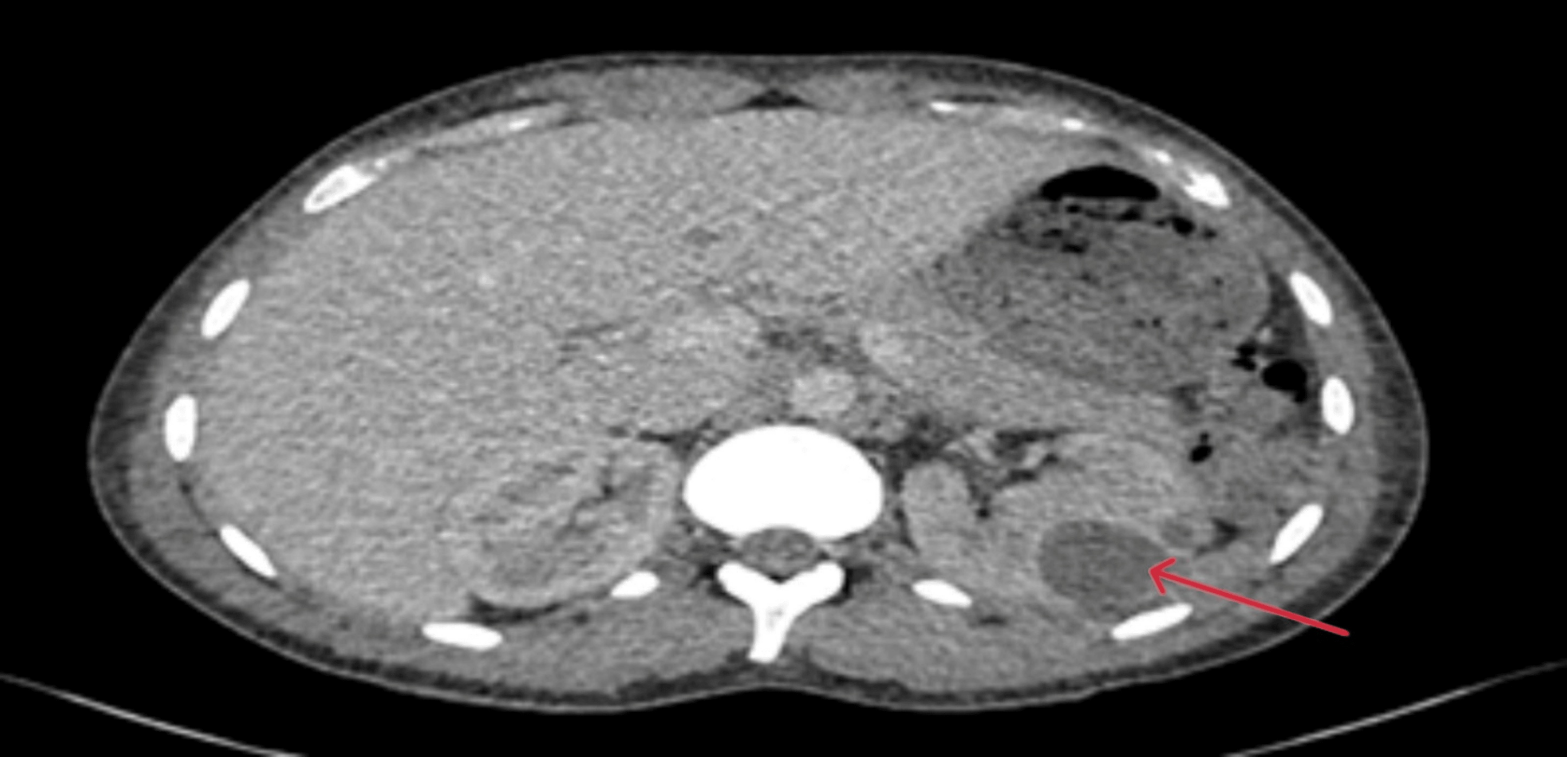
A CT abdomen on Day 12 demonstrated the interval development of a 3.5 cm renal abscess in the left interpolar region. There also remains a slightly loculated left subcapsular collection, which measures up to 1.4 cm in depth. No hydroureteronephrosis bilaterally. The red arrow indicates a renal abscess in the left interpolar region.
